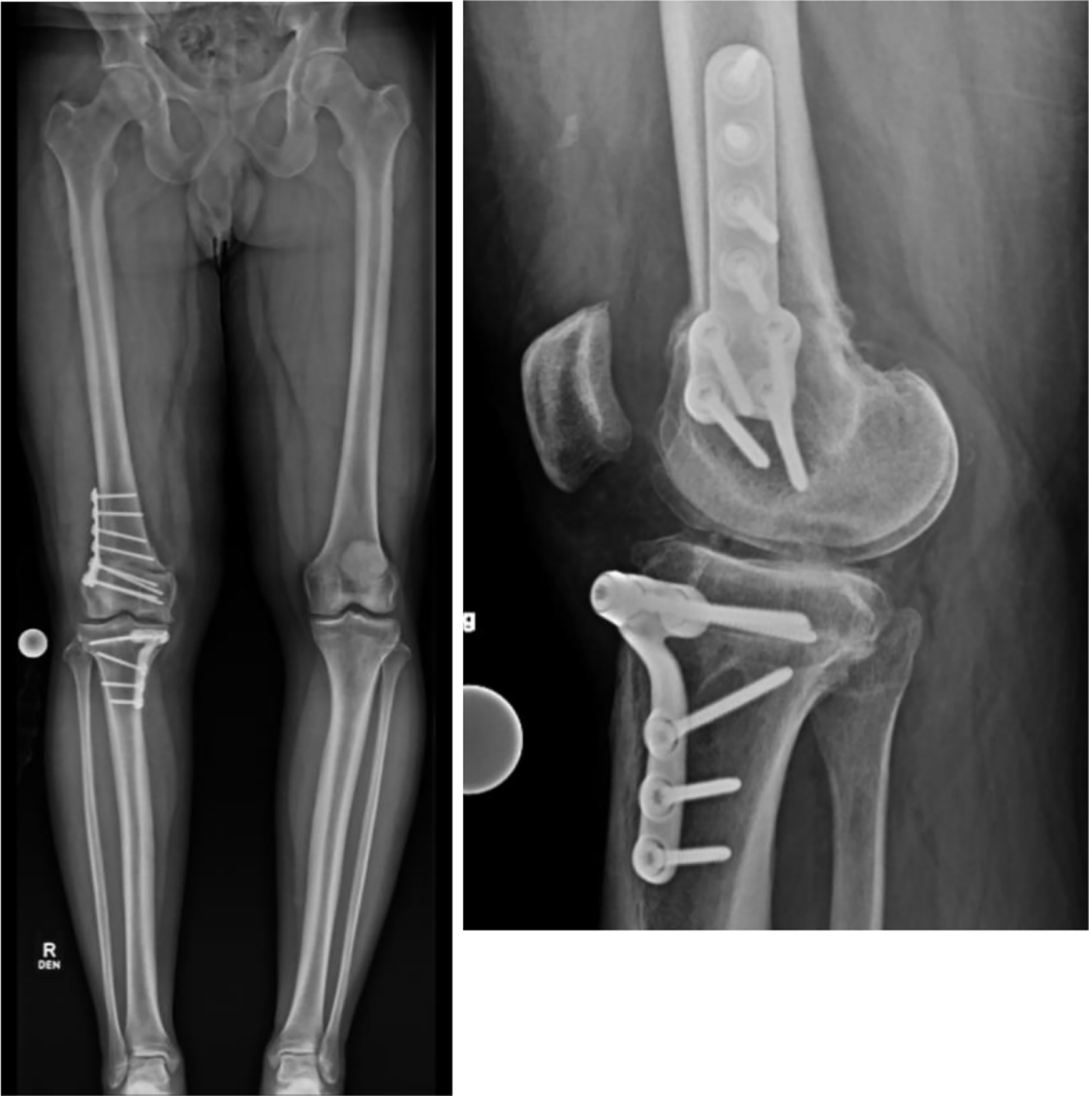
Final full-length anteroposterior and lateral radiographs of the patient from Figs. 1 and 3 months post-operatively
